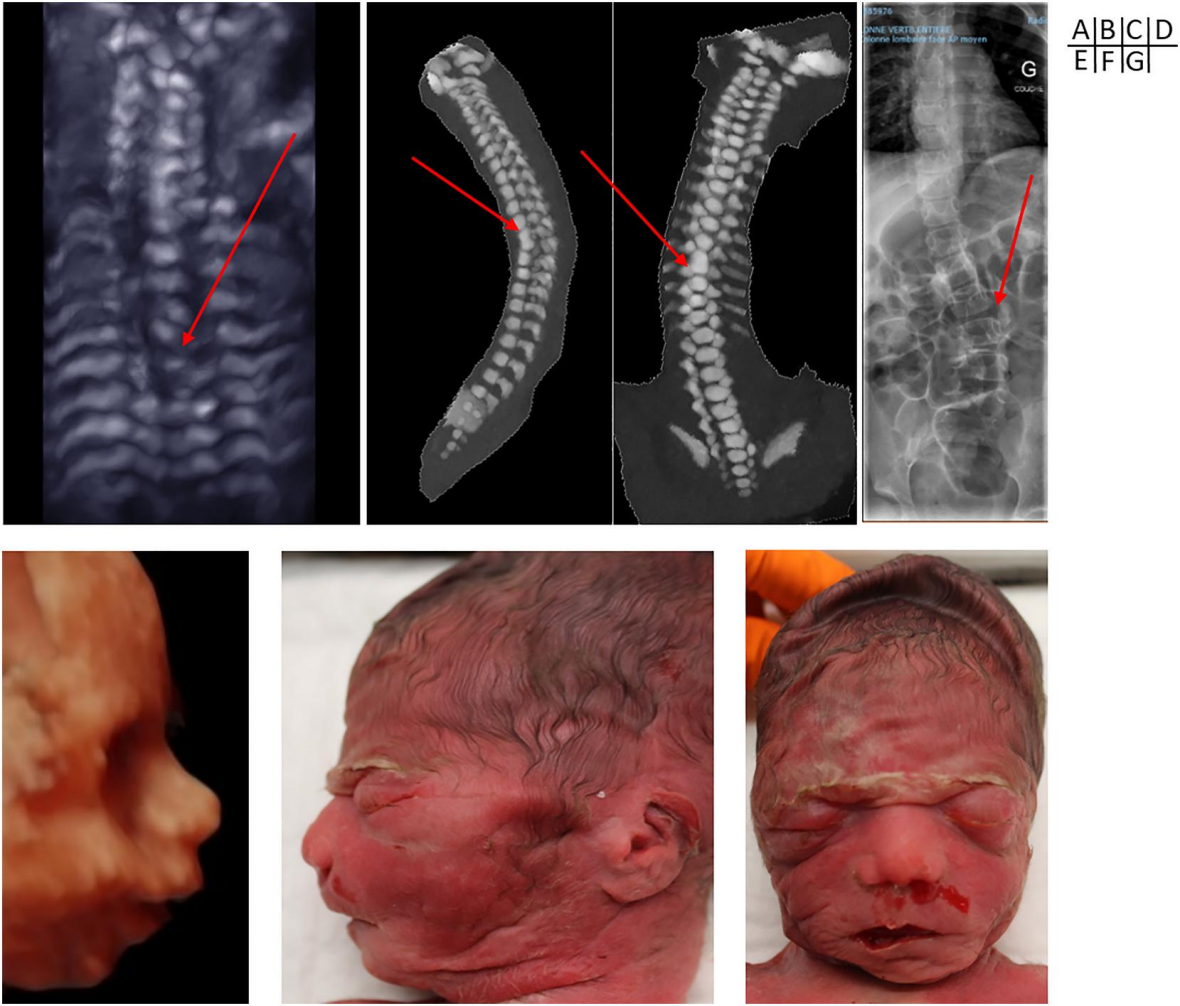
Prenatal imaging and postnatal appearance of other anomalies associated with Aicardi syndrome (A–D) Prenatal ultrasound (3D bone rendering), prenatal computed tomography, and postnatal X‐rays showing thoracic scoliosis with hemi‐vertebrae (A and D) or vertebral bloc (B and C) in Cases 2, 5, and 19 (courtesy of Léo Pomar and Laurent Guibaud) (E–G) Prenatal ultrasound (3D surface) and post‐mortem aspect showing facial dysmorphism (bulging premaxilla, upturned nostrils, retrognathia) in Case 3 (courtesy of Léo Pomar and Estelle Dubruc)
